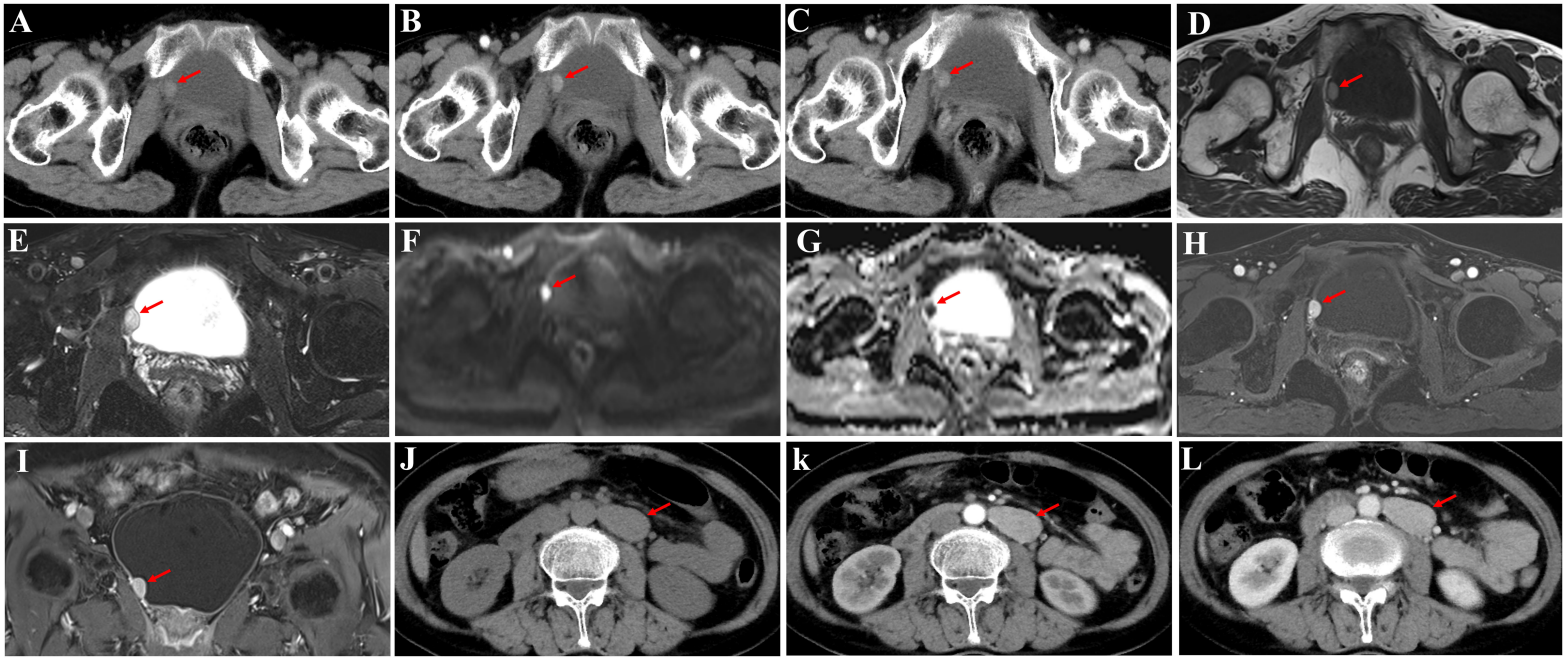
Imaging findings of a 59–year–old female patient. Bladder wall nodule (A–I) and left retroperitoneal tumor (J–L). (A) A well–defined, round soft tissue nodule located on the right bladder wall, growing into the lumen, with smooth margins and homogeneous density on non–contrast CT (attenuation value: 55 HU). (B) Arterial–phase contrast–enhanced CT showing an attenuation value of 78 HU. (C) Venous–phase contrast–enhanced CT showing an attenuation value of 90 HU. (D, E) The lesion exhibits slightly high signal intensity on T1WI and fat–saturated T2WI, with a homogeneous signal pattern. (F, G) DWI shows high signal intensity, while the ADC demonstrates low signal intensity. (H, I) The lesion exhibits significant and persistent enhancement on contrast–enhanced imaging. (J) A well–defined, round soft tissue mass in the left retroperitoneum, with smooth margins and homogeneous density on non–contrast CT (attenuation value: 48 HU). (K) Arterial–phase contrast–enhanced CT showing an attenuation value of 97 HU. (L) Venous–phase contrast–enhanced CT showing an attenuation value of 110 HU. Arrow, lesion location. CT, computed tomography, T1WI, T1–weighted image, T2WI, T2–weighted image, DWI, diffusion weighted imaging, ADC, Apparent diffusion coefficient.
